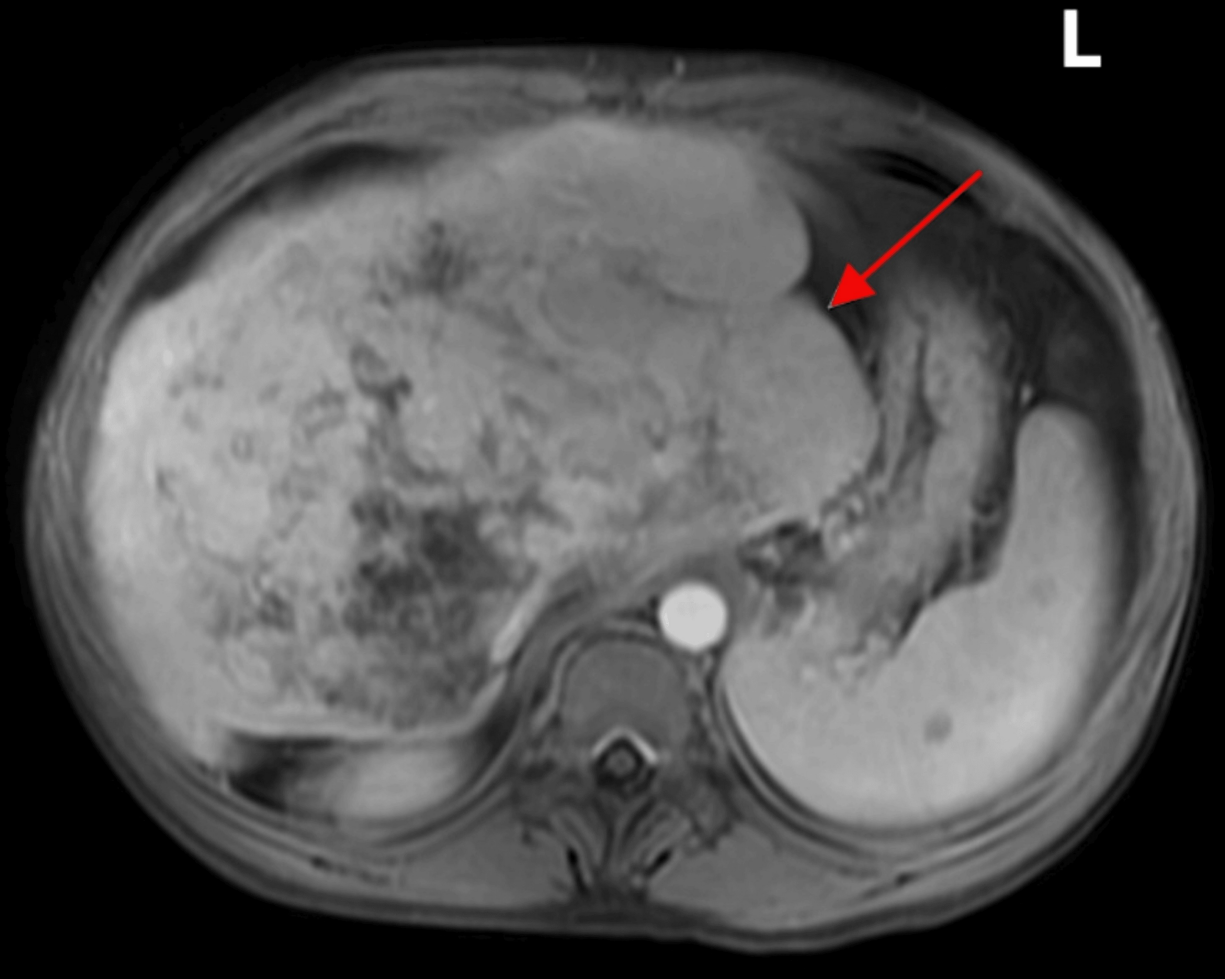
Axial MRI (T1-weighted water image) of the upper abdomen performed with gadolinium contrast, showing marked hepatomegaly with heterogeneous signal intensity throughout the liver. The red arrow points to the markedly enlarged caudate lobe of the liver, a characteristic finding in chronic Budd-Chiari syndrome. The central liver parenchyma shows architectural distortion and progressive contrast enhancement, consistent with fibrosis and venous outflow obstruction. No focal discernible liver lesion was identified on the MRI to suggest malignancy. ‘L’ indicates the left side of the body.
